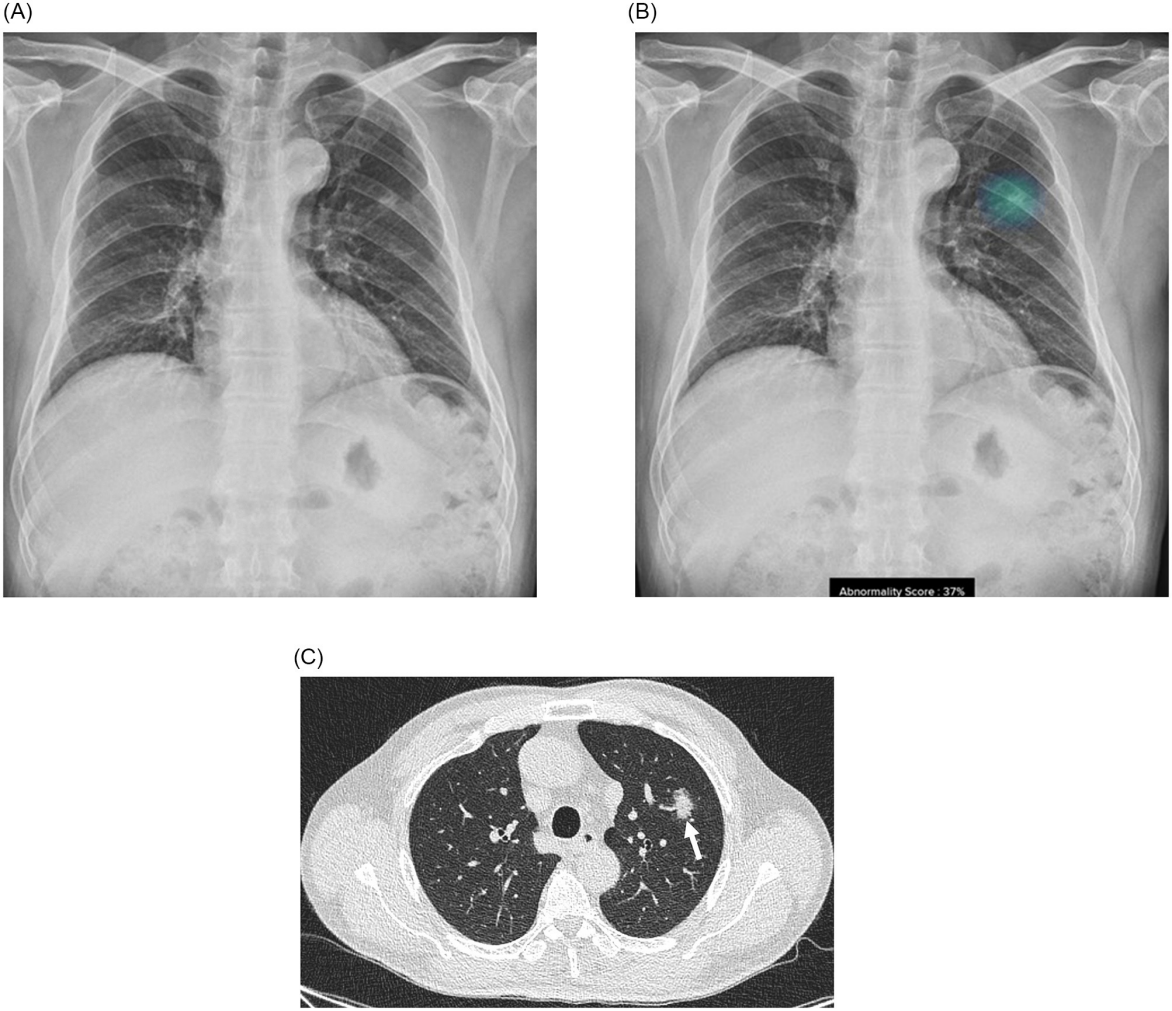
Chest radiograph and computed tomography (CT) of a 60-year-old man (#2 patient). (A) Chest radiograph was taken before performing cholecystectomy for chronic cholecystitis. (B) Artificial intelligence-based lesion detection software detected a lung nodule in the left upper lobe with an abnormality score of 37%. (C) After cholecystectomy, chest CT demonstrated a part-solid nodule in the left upper lobe (arrow), and pathology confirmed adenocarcinoma after surgical resection (total tumor size of 2.3 cm; invasive size of 0.75 cm). The final stage was IA1 (pT1aN0M0). The patient is now undergoing routine follow-up in the outpatient clinic without additional treatment.
